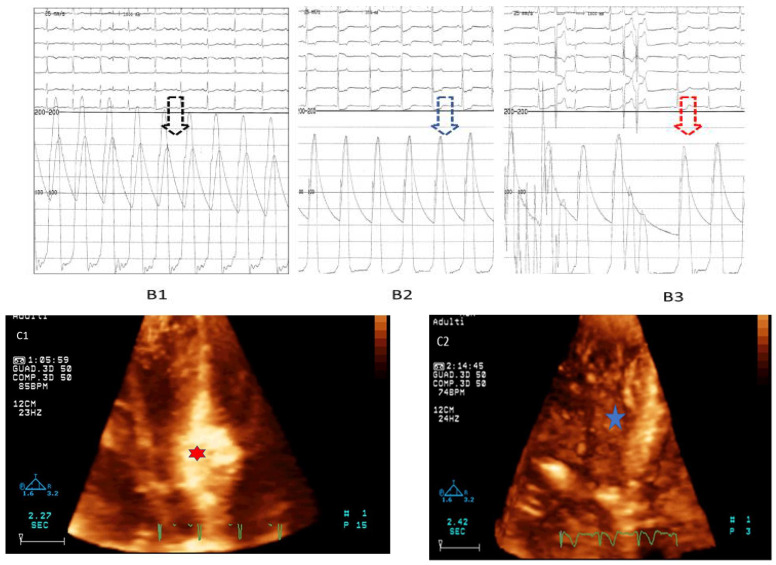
Successful alcohol septal ablation. (A1): 3D-TTE showing the septal contact of systolic anterior motion of mitral valve (blue arrow) (target septal zone: blue star); (A2): Cannulation of the first coronary septal branch (red arrow) with subsequent opacification of target septal zone (blue star) at MC-3DE following intracoronary echo-contrast injection (A3); (B1–B3): Intraprocedural hemodynamic monitoring during alcohol injection into the first coronary septal branch with disappearance of baseline intraventricular gradient ((B1), dashed black arrow) following the last alcohol injection ((B2), dashed blue arrow) without inducibility during the post-extrasystolic ventricular beat ((B3), dashed red arrow); (C1): 3D-TTE showing target septal zone opacification (red star) following alcohol injection; (C2): 3D-TTE at one-year follow-up showing optimal remodeling of target septal zone (blue star) without dynamic obstruction. 3D-TTE: three-dimensional transthoracic echocardiography; MCE: myocardial contrast three-dimensional transthoracic echocardiography.
